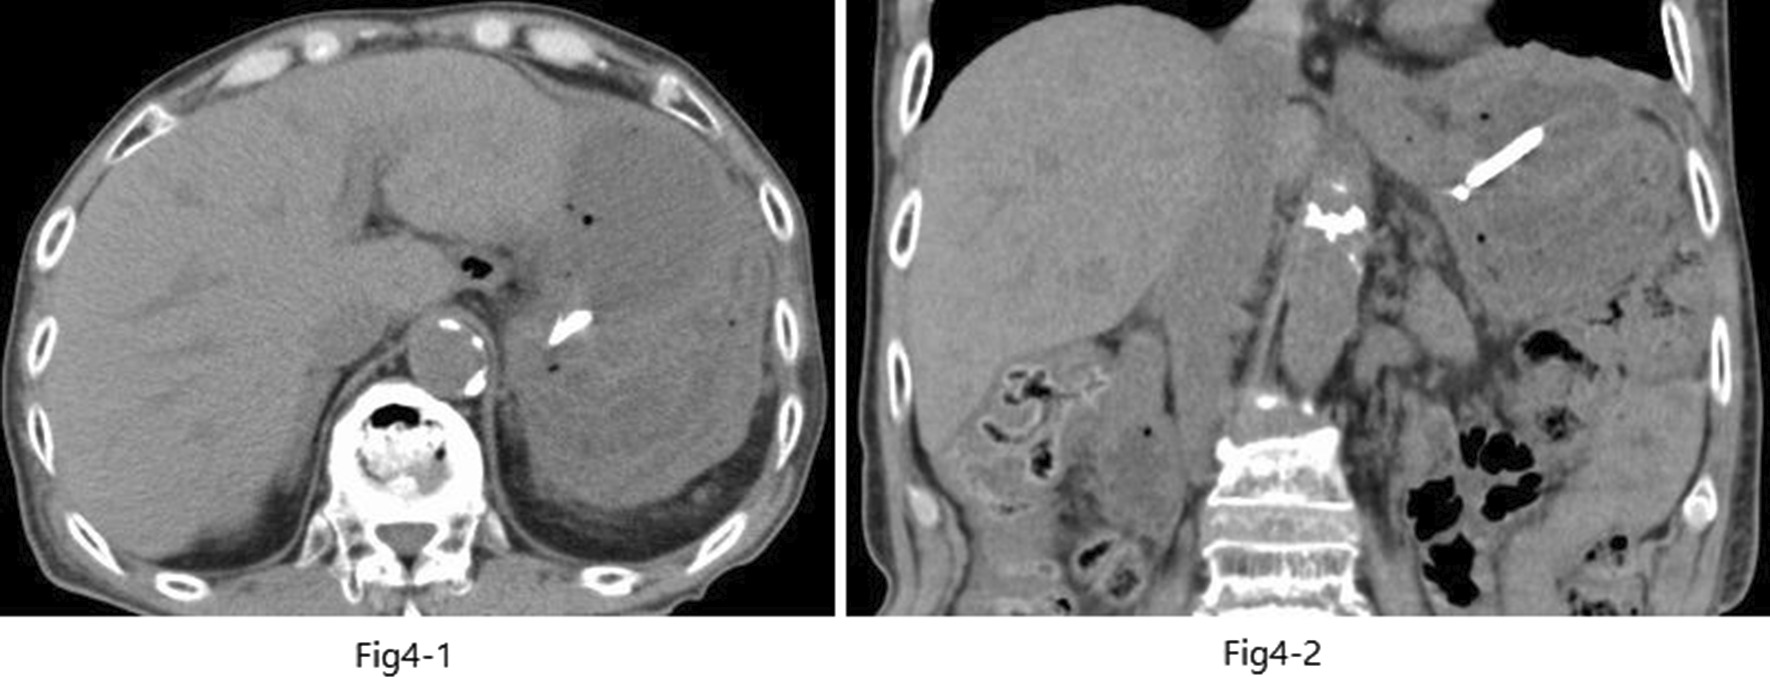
(1) Computed tomography scan (coronal view) confirming the drain (white line) placed in the abscess. (2) Computed tomography scan (axial view of the same image as in 1)
